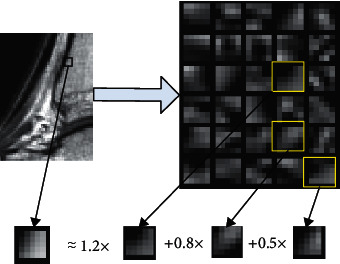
Sparse representation of MR image blocks.
